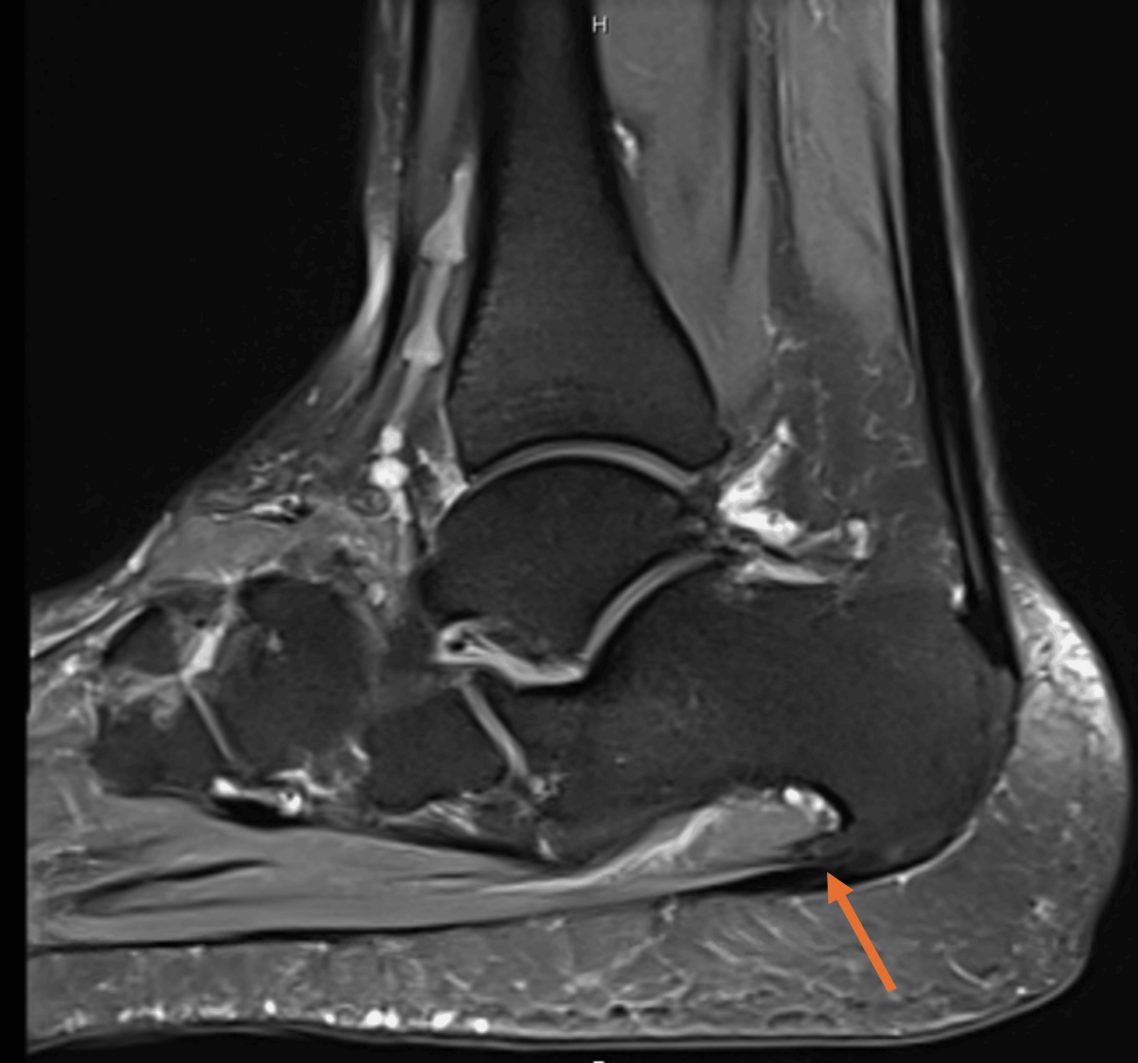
Proton density sagittal-weighted MRI revealing calcaneal spur, plantar fasciitis, and Achilles tendinopathy (arrows) MRI: magnetic resonance imaging
